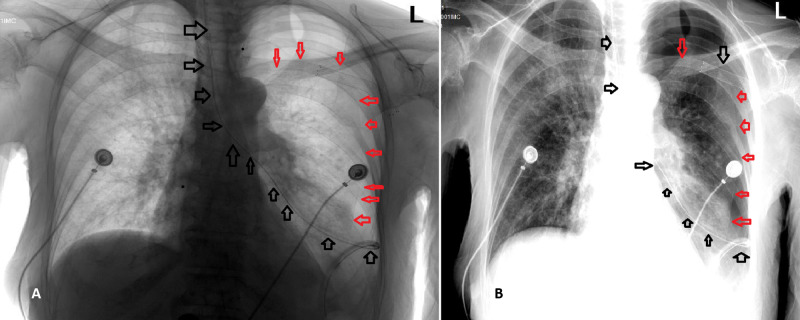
CX-ray immediately after the incorrect placement of NGT Image A shows the inverse mode, and image B shows the regular mode of CX-ray immediately after the incorrect placement of NGT (black arrows), and the development of left-sided PTX (red arrows). CX-ray, chest X-ray; NGT, nasogastric tube; PTX, pneumothorax.
